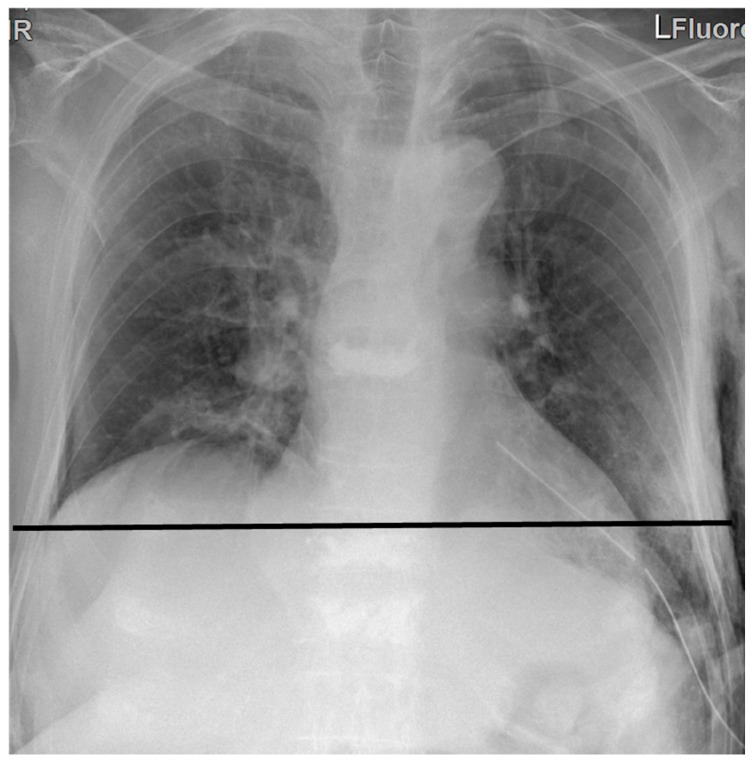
Postoperative chest X-ray after plication of the left side of the diaphragm. The black line demonstrates the new level after the plication of the left cupola.
